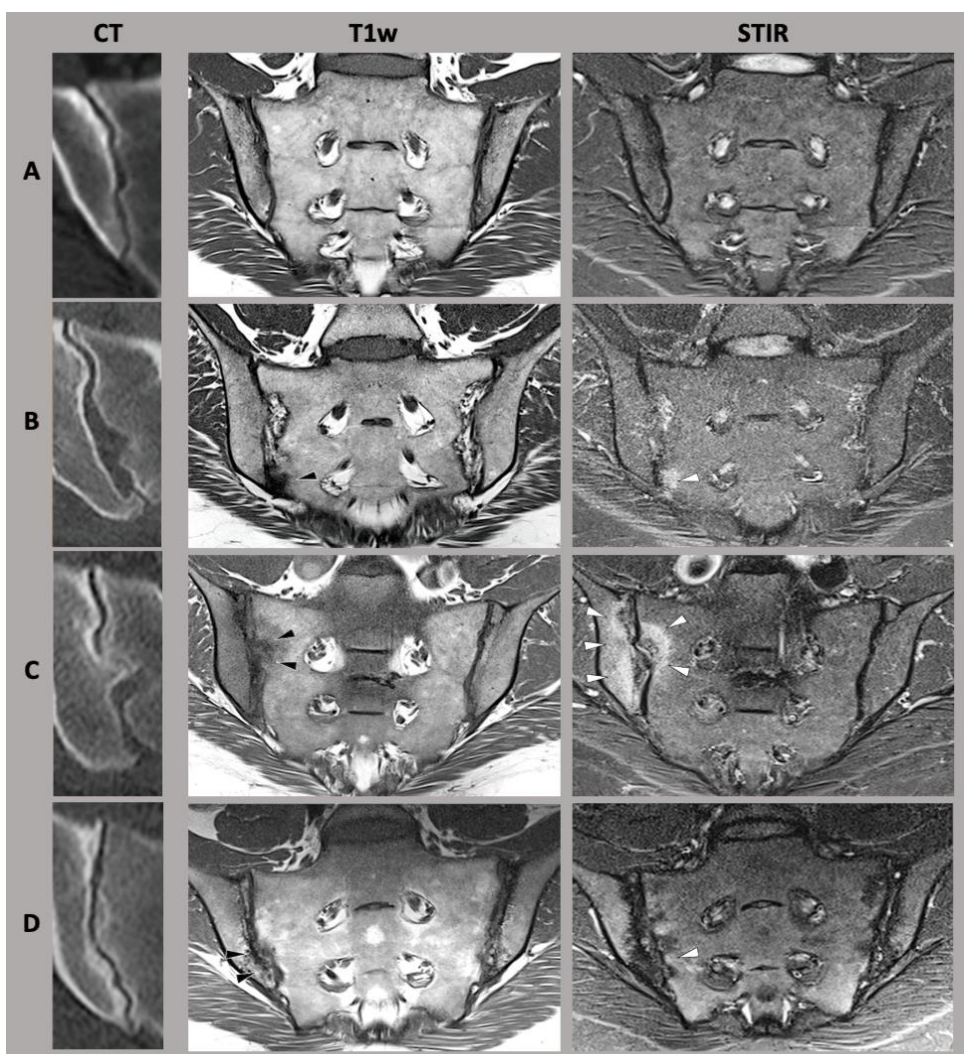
A selection of sacroiliac joint form variation. (CT) computed tomography, (T1w) T1-weighted magnetic resonance imaging sequence, (STIR) short-tau inversion recovery magnetic resonance imaging sequence. (A) typical sacroiliac joint form, (B) accessory joint facet, (C) sacroiliac complex, (D) crescent-shaped ilium. Adapted with permission from Refs. [6,24]. Copyright 2021-22, copyright Katharina Ziegeler.
